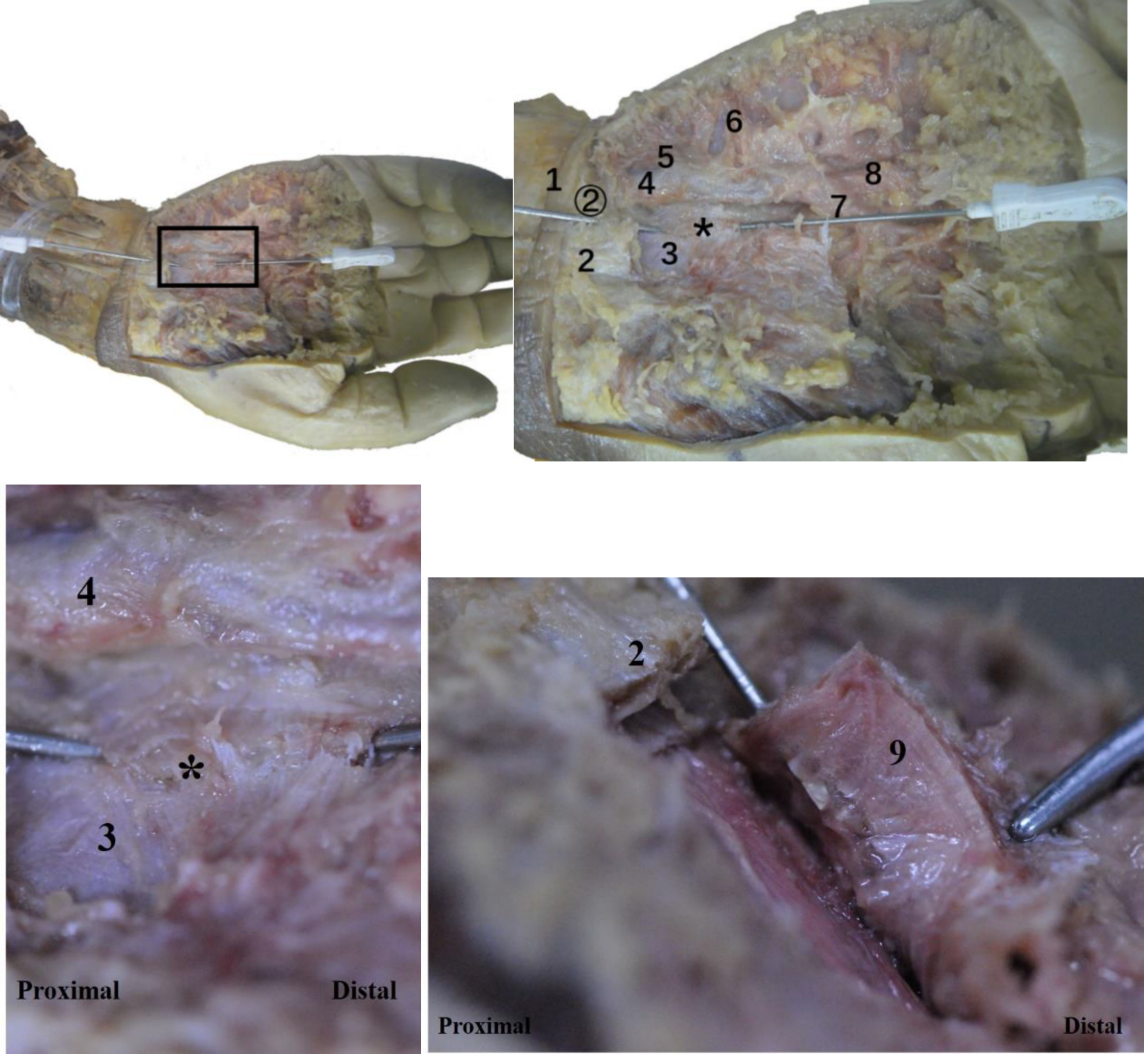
The vertical view (top left, top right) and side view (bottom left, bottom right) of the ultrasonic guided needle knife cutting trace. The asterisk refers to the cutting marks of the needle knife. 1. Proximal transverse carpal striae; 2. Palmar longus tendon connected to the palmar aponeurosis (cut); 3. Transverse carpal ligament; 4. Ulnar artery; 5. Ulnar nerve; 6. Superficial palmar branch of ulnar artery; 7. Palm Superficial arch; 8, common palmar artery; 9, transverse carpal ligament (turned up); ②, needle entry point 2.
